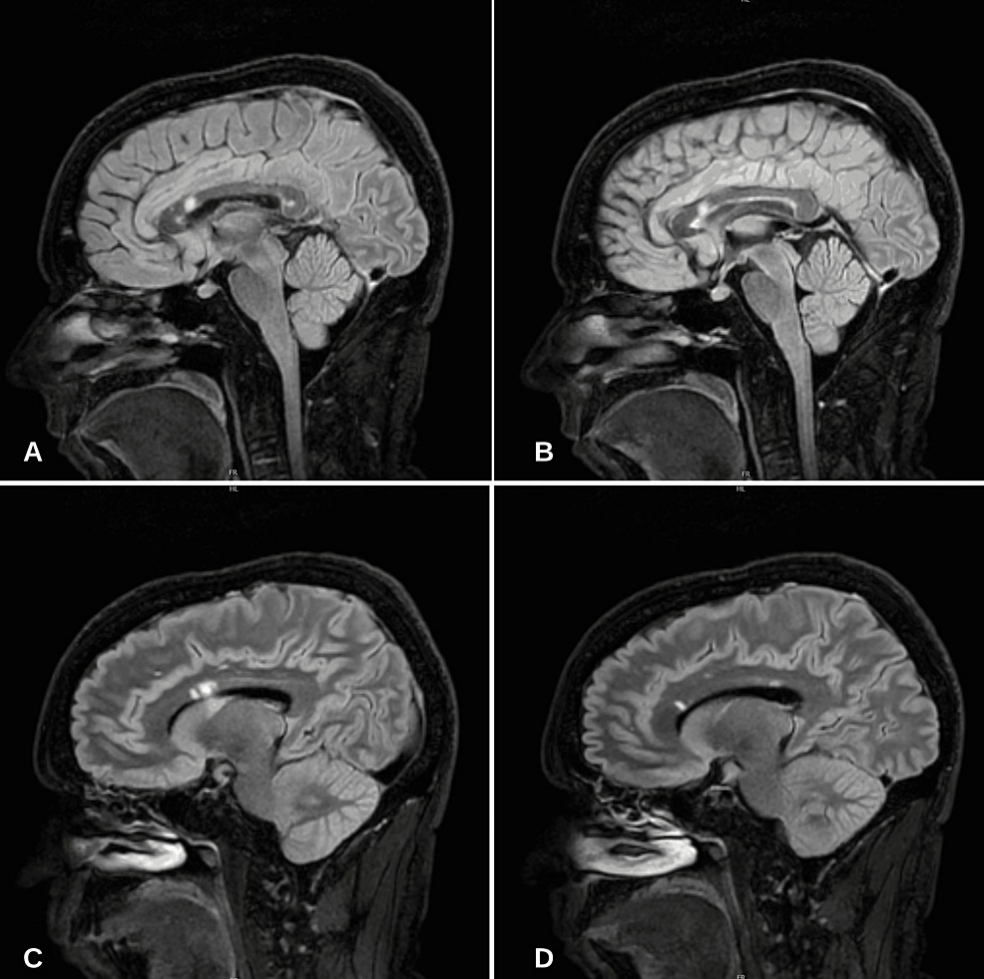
MRI T2-sagittal view revealing multiple small-to-large white matter lesions ("snowballs") and linear lesions ("spokes") A-C: Multiple large white matter lesions (snowballs) seen within the rostrum, genu, and body of the corpus callosum. D: Linear (spoke) lesion seen within the genu of the corpus callosum.
